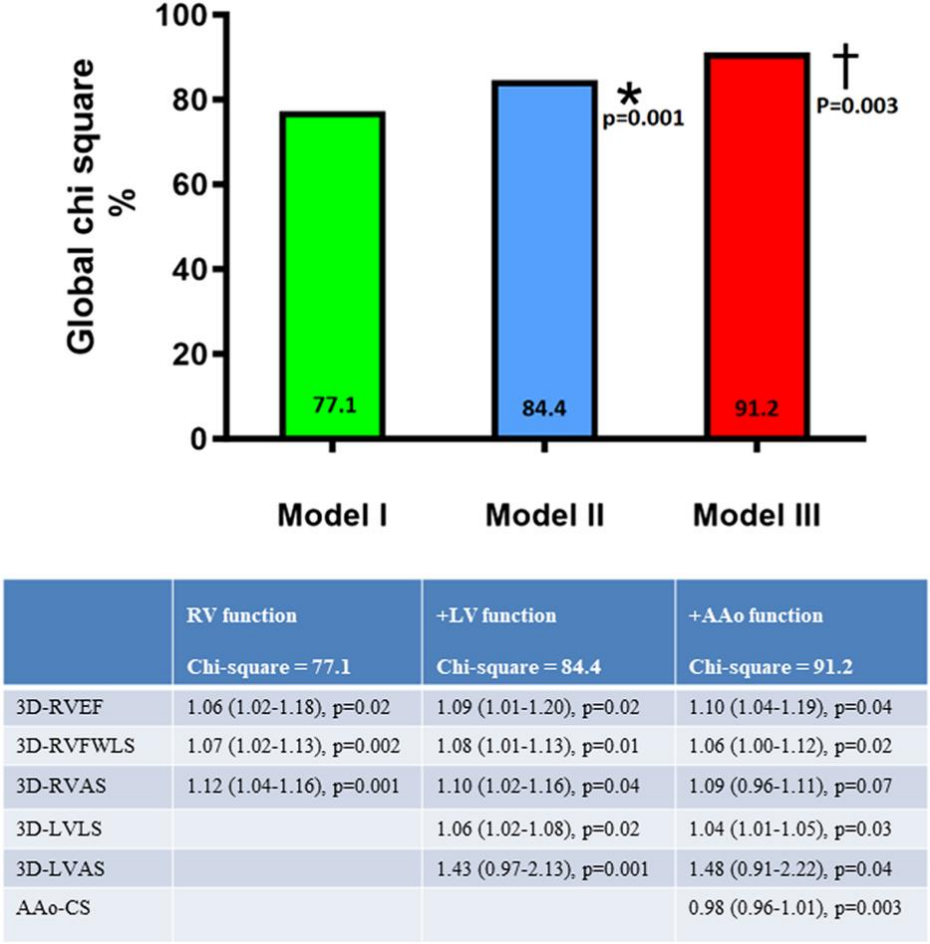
Incremental value of additional LV and AAo dysfunction parameters over standalone RV echocardiographic variables in predicting exercise capacity impairment. Model 1 included RV-STE parameters (3D-RVEF < 48%, 3D-RVFWLS > −22%, and 3DRVAS > −25.5%). Model 2 included RV dysfunction + LV dysfunction parameters (3DLVLS > −20% and 3DLVAS > −30.2%). Model 3 included RV strain + LV strain + AAo dysfunction parameters (AAo-CS < 7.5%). *Model 1 vs. Model 2: *χ*^2^ values 77.1 vs. 84.4, *P* = 0.001; †Model 2 vs. Model 3: *χ*^2^ values 84.4 vs. 91.2, *P* = 0.003. 3D, three-dimensional; LVAS, left ventricular area strain; LVLS, left ventricular longitudinal strain; RVAS, right ventricular global area strain; RVEF, right ventricular ejection fraction; RVAS, right ventricular area strain; RVFWLS, right ventricular free-wall longitudinal strain.
